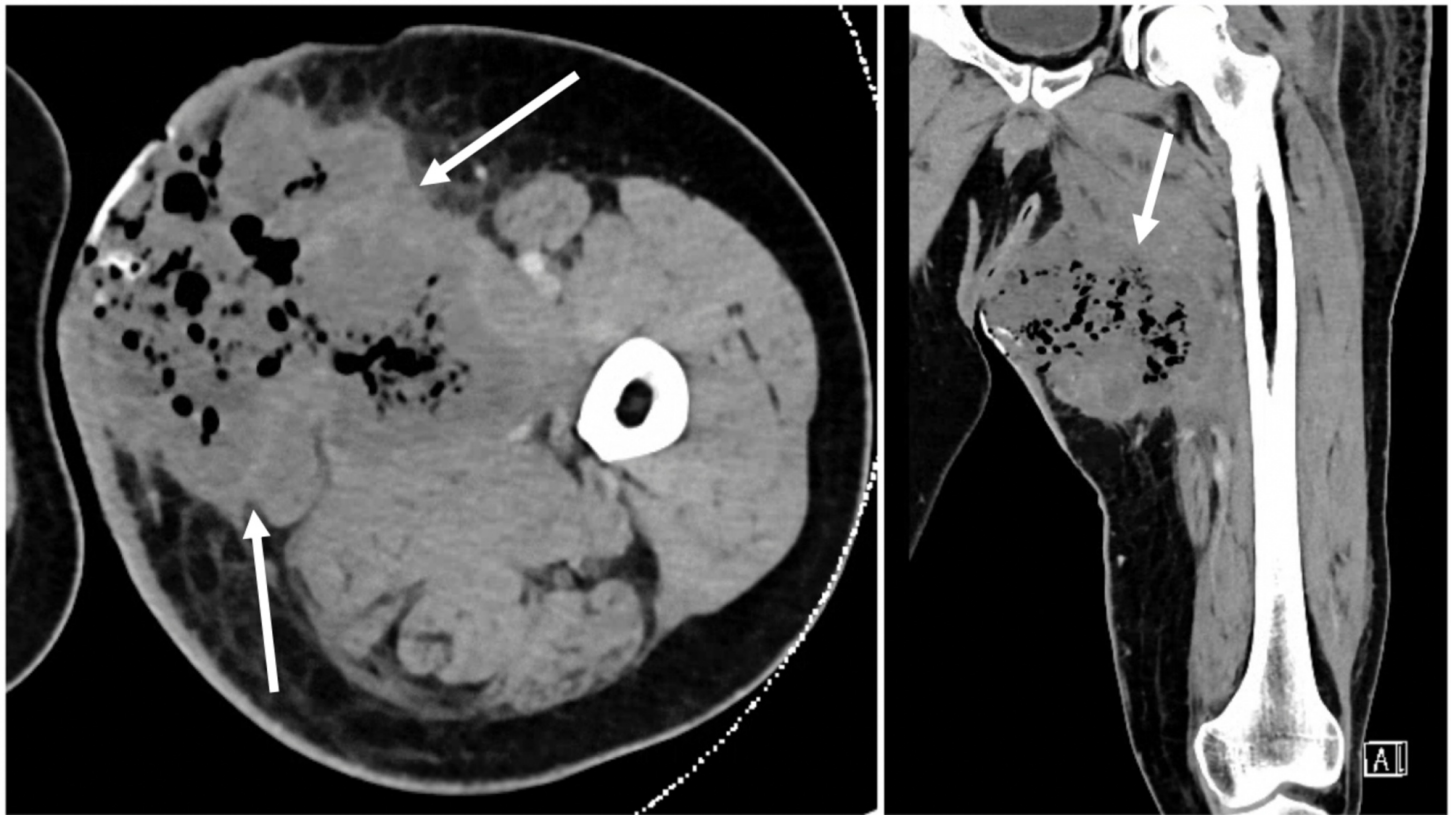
(Left to right: axial view, coronal view) CT scan showing 8.6 x 8.4 x 11.4 cm large, medial proximal thigh soft tissue mass with intralesional gas due to tissue necrosis (white arrows) CT: computed tomography
